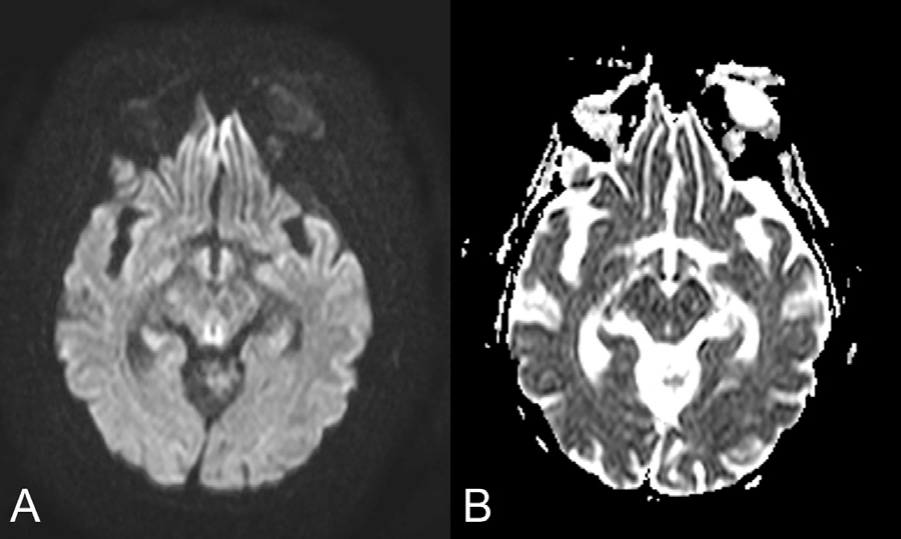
Axial diffusion-weighted imaging b-1000 (DWI) shows restricted diffusion, with high signal intensity in the tectal plate (A) and reduced intensity on ADC values (B).
